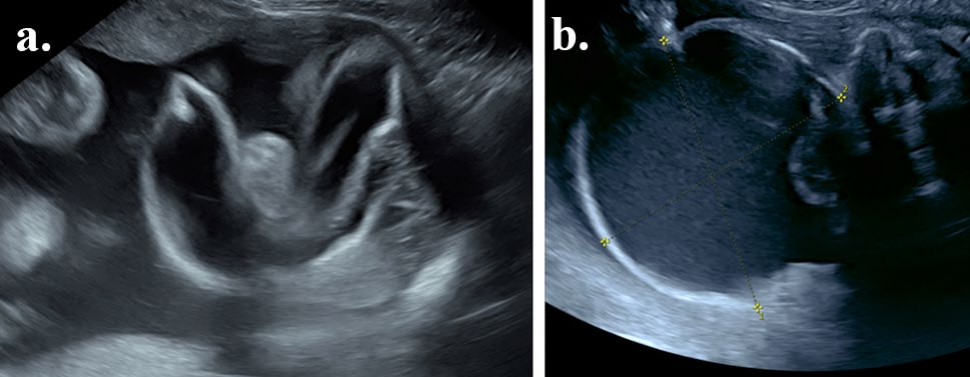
Ultrasound images of the lesion before and after drainage. a highlights the collapse of the cystic structure after the removal of 640 ml of light yellow coloured at 33 + 3 weeks gestation. b shows the reaccumulation by 34 + 3 weeks gestation
